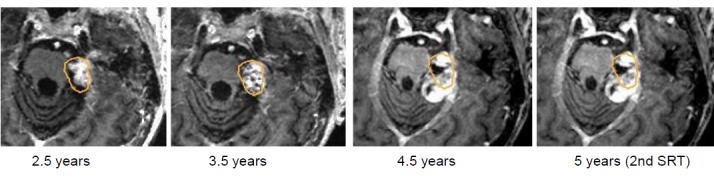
Best clinical condition and MRI findings up to 2.5 years after irradiation further marginal tumor regression. 4 years later, again significant tumor growth to 14.2 cm³. Meanwhile, the patient had to undergo dialysis because of renal failure. She strictly refused surgery. Upon her urgent wish, again SRT with 22x1.8 Gy was started that had to be interrupted after the 16th fraction because of a fall with bilateral fractures of the ischium and the pubic bones and intensive care that was necessary because of metabolic imbalance. The patient dies of multiorgan failure.
